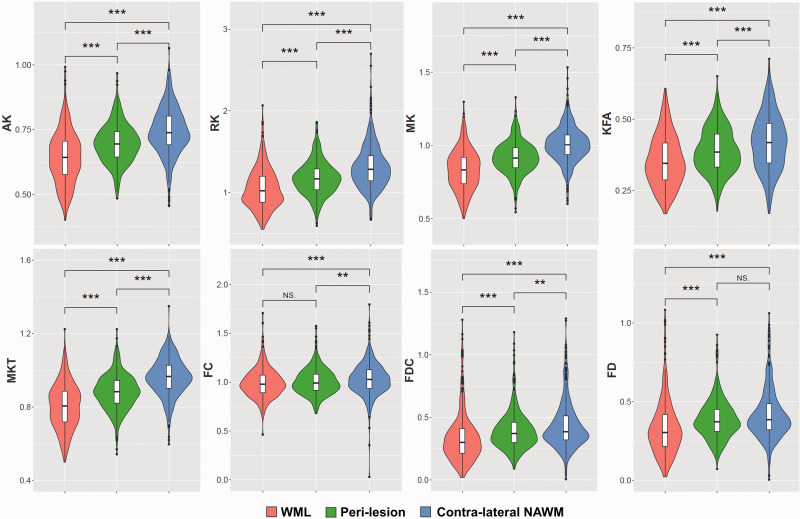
Kurtosis and fixel-based diffusion MRI parameters.
